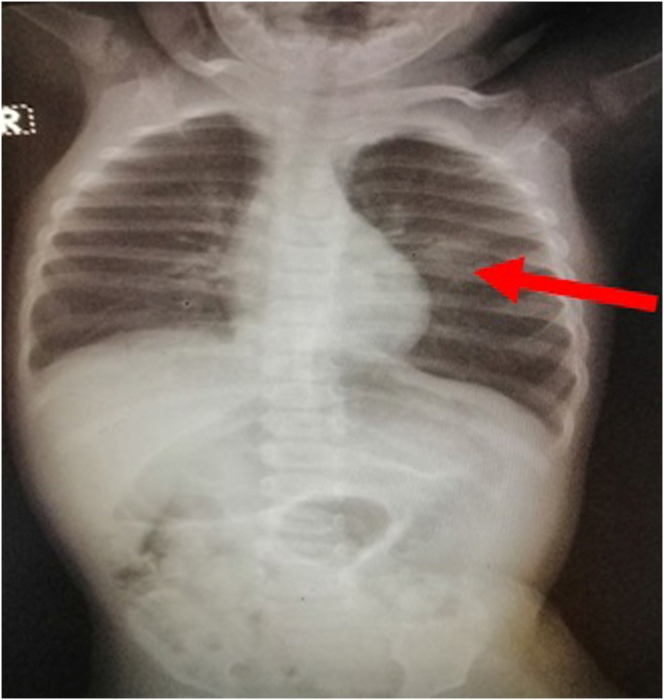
Patient's chest radiography. A round-shaped opacity in the left lung could be noted (arrow).
